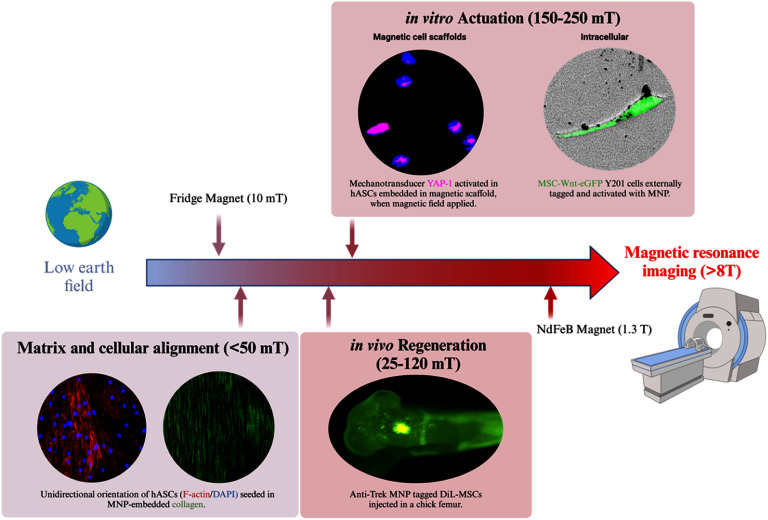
Applications of magnetic fields in biomedical engineering. Low flux density magnetic fields (<50 mT) can align MNP-tagged cells or matrix proteins. Increasing magnetic flux densities (25–250 mT) can initiate magnetic actuation of cells *in vitro* and *in vivo* for regenerative medicine applications. Higher magnetic flux densities (>8 T) are often required for magnetic resonance imaging. Created with BioRender.com.
